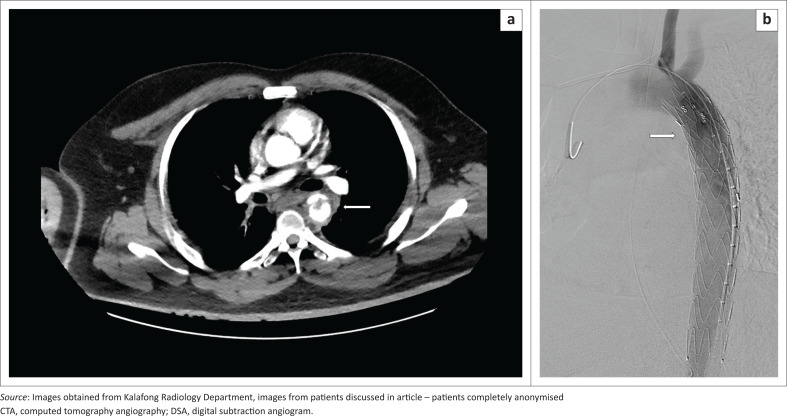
(a) A 45-year-old male jumped from the fourth floor and sustained multiple injuries. Initial computed tomography angiography revealed a traumatic type 2 aortic injury with an intimal flap, thrombus and false aneurysm (arrow). (b) Sagittal oblique digital subtraction angiogram image with the stent in place (arrow). No complications seen.
